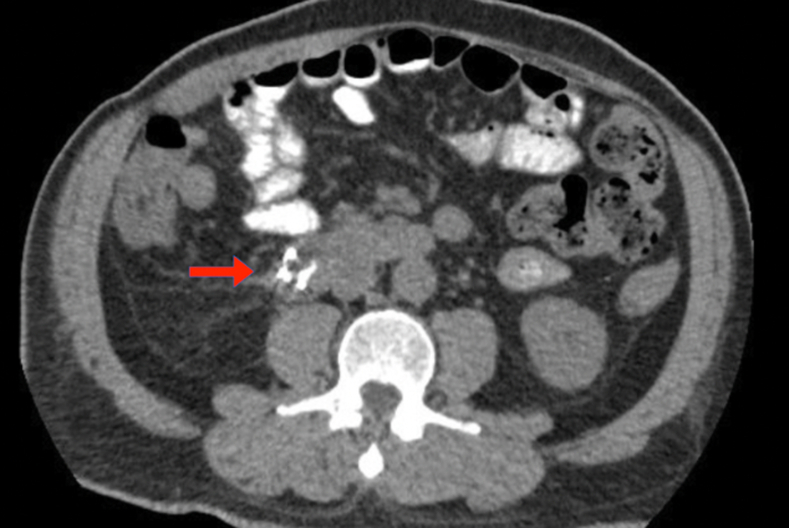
A postprocedural CT scan showed the clip in the proper place and no extraluminal fluid collection, air, or contrast extravasation.
